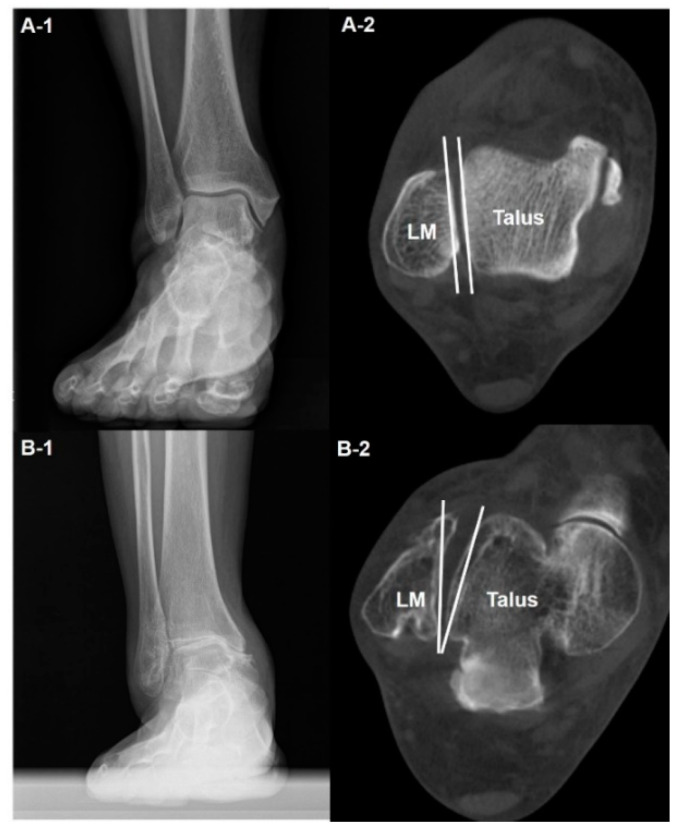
Abnormal internal rotation of talus in the axial plane in varus ankle arthritis. Plain weight-bearing anteroposterior ankle radiography of the normal ankle (A-1) with corresponding WBCT axial image showing the congruent position of the talus in the ankle joint, without rotation against the lateral malleolus (LM) (A-2). Plain weight-bearing anteroposterior ankle radiography of a patient with varus ankle osteoarthritis (B-1), with WBCT axial image showing the internally rotated talus against the lateral malleolus (LM) (B-2).
